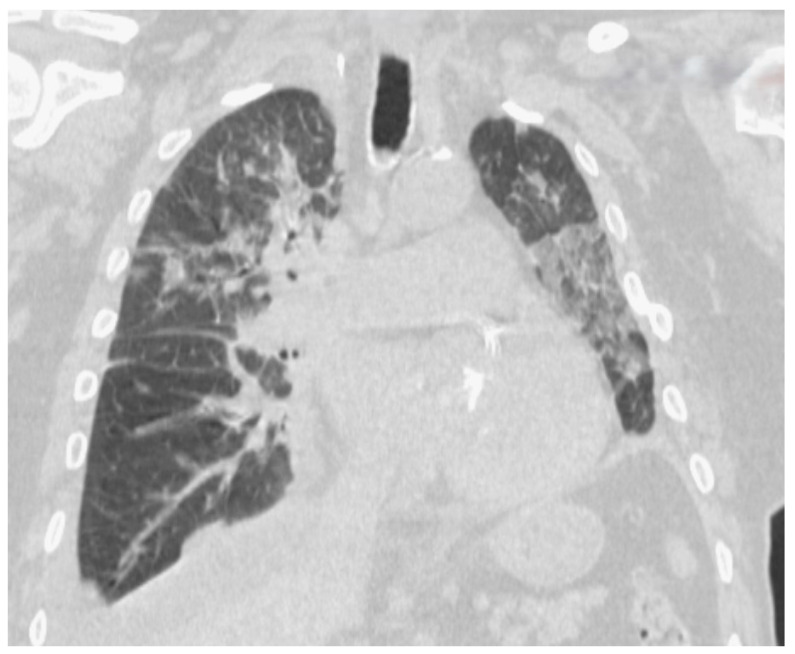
Chest CT—diffuse left and right superior lobe alveolar ground-glass opacities with crazy-paving pattern and bilateral pleural effusion.
